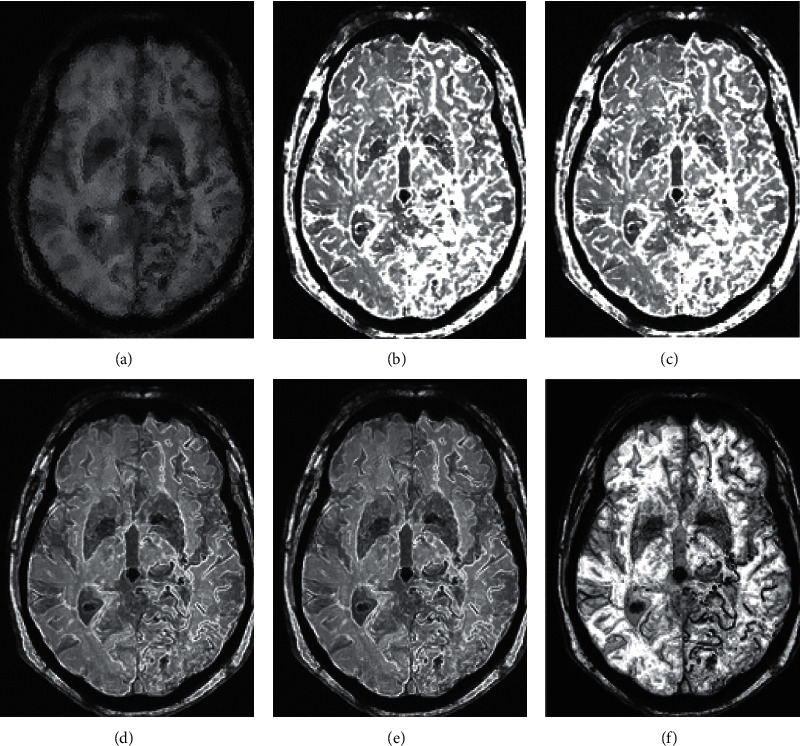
GMM-based clustering results of MRI images of stroke patients. *Note.* Figures (a), (b), (c), (d), (e), and (f) were images with 10% noise; clustering results of noisy images; clustering results generated by iterations; clustering results after 3 circles of iterations; clustering results after 6 circles of iterations and the denoised image.
